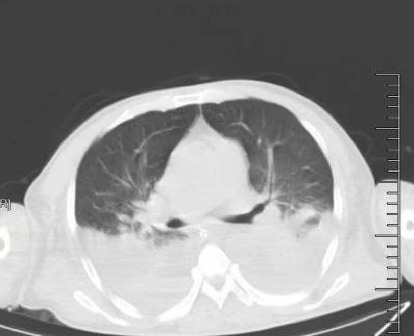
Chest CT: bilateral pleural cavities with a moderate amount of effusion and incomplete expansion of adjacent lung tissue.
